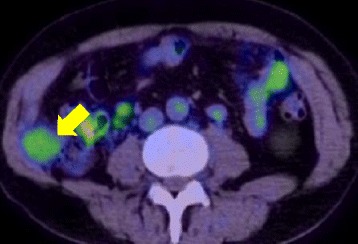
Positron emission tomography-computed tomography image of the mass (*arrow*)
